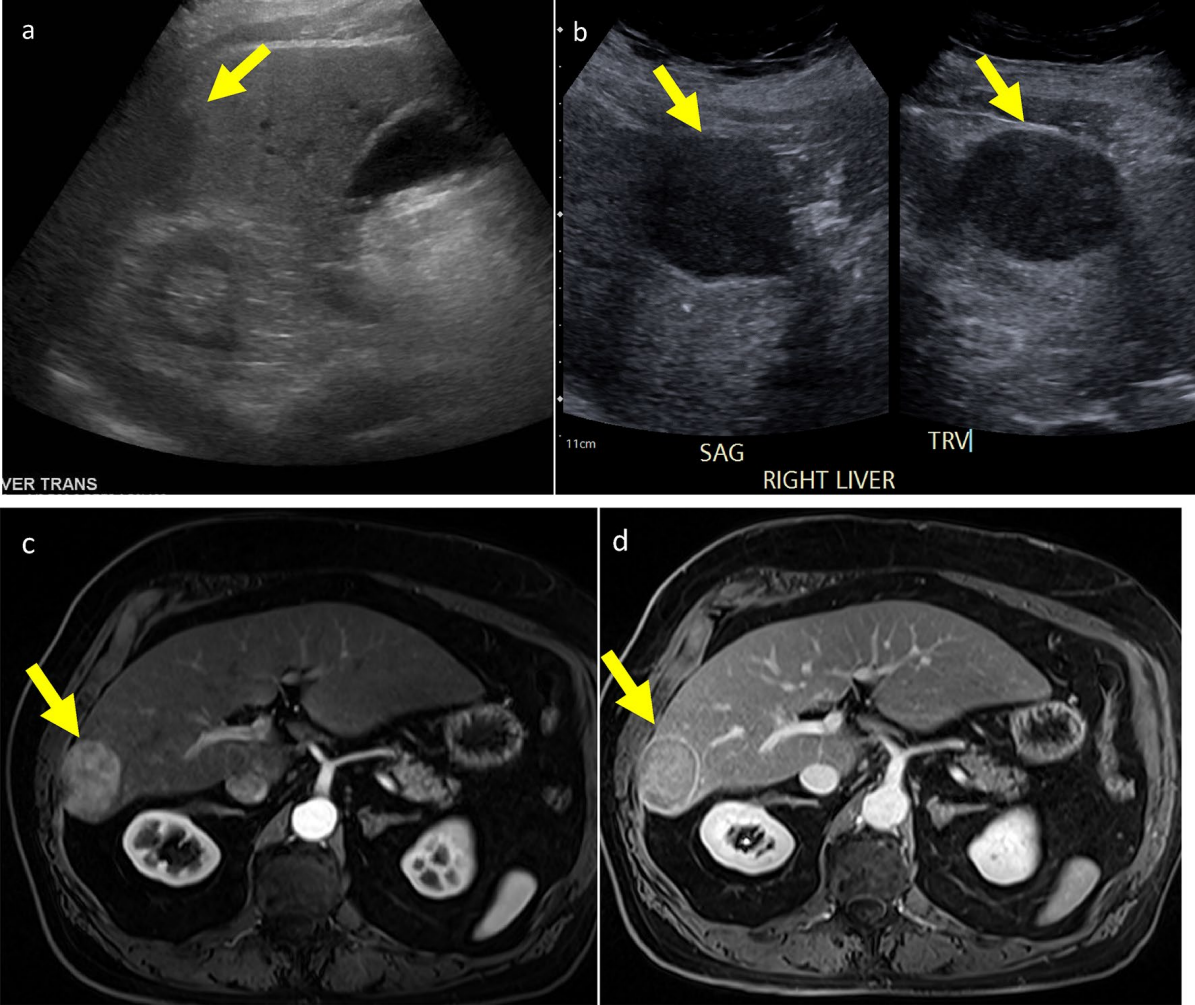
Liver mass initially not seen on routine abdominal US performed in a 63-year-old male with metabolic dysfunction-associated steatohepatitis (MASH) and F3 fibrosis. a Transverse grayscale US image right lobe of the liver shows a peripheral hypoechoic region (arrows), only visualized on retrospective review of the images, that may correspond to the observation detected in b. b Patient returned for US elastography 3 weeks later, and sonographer placed patient in a decubitus position. Sagittal and transverse grayscale US dual screen image of the liver shows a 4.6 cm solid hypoechoic mass (arrows). This case underscores the need to examine the liver’s periphery, which can be enhanced by placing the patient in a decubitus position, suspending respiration, and using intercostal and subcostal scanning. c and d Axial contrast-enhanced MRI of the abdomen in the arterial (c) and delayed (d) phases show arterial phase hyperenhancement of the observation with ‘washout’ and enhancing ‘capsule’, representing HCC
